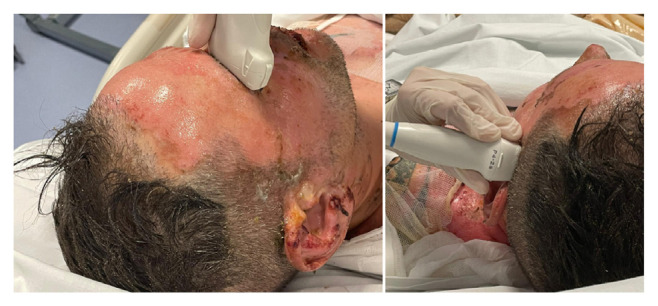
Two ONSD (left) and TCCD (right) measurement images on the head of a severely burned patient (80% extension) with “accessible ultrasound windows”, as they are not affected by the burn.
